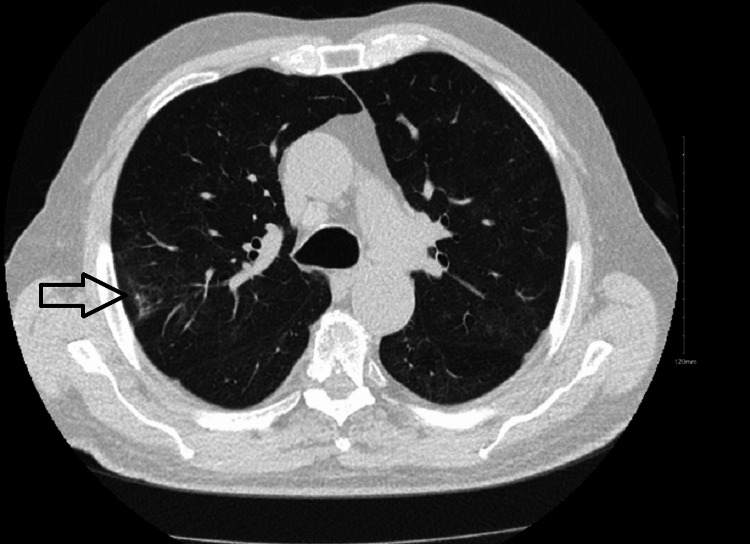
Computed tomography of the chest without contrast showing mild emphysematous changes with prominent interstitial markings in the right lobe (right arrow).
